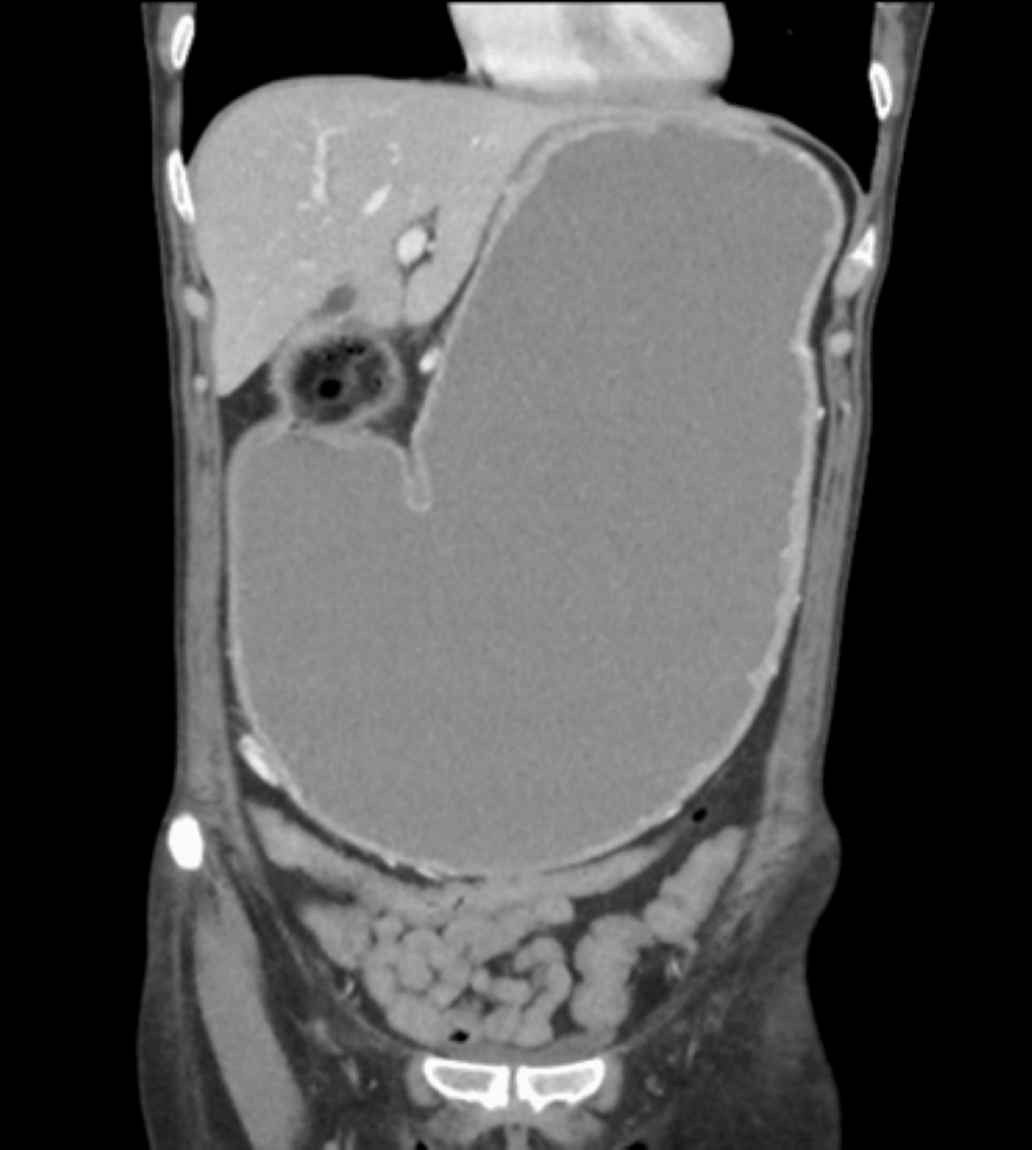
CT scan of the thorax, abdomen, and pelvis in coronal view CT showing marked distension of the stomach, with features highly suggestive of gastric outlet obstruction due to an atypical pyloric circular ulcer with rolled edges. CT: Computed tomography
